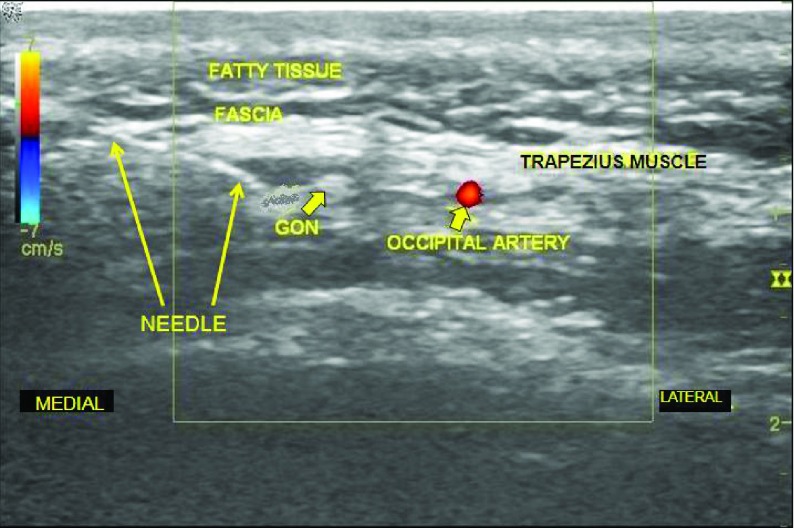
Ultrasound Image Showing the Needle Adjacent to the Greater Occipital Nerve Immediately Before Injection of Local Anesthetic/Steroid Mixture During Ultrasound-Guided Diagnostic Greater Occipital Nerve Block. GON = Greater Occipital Nerve
